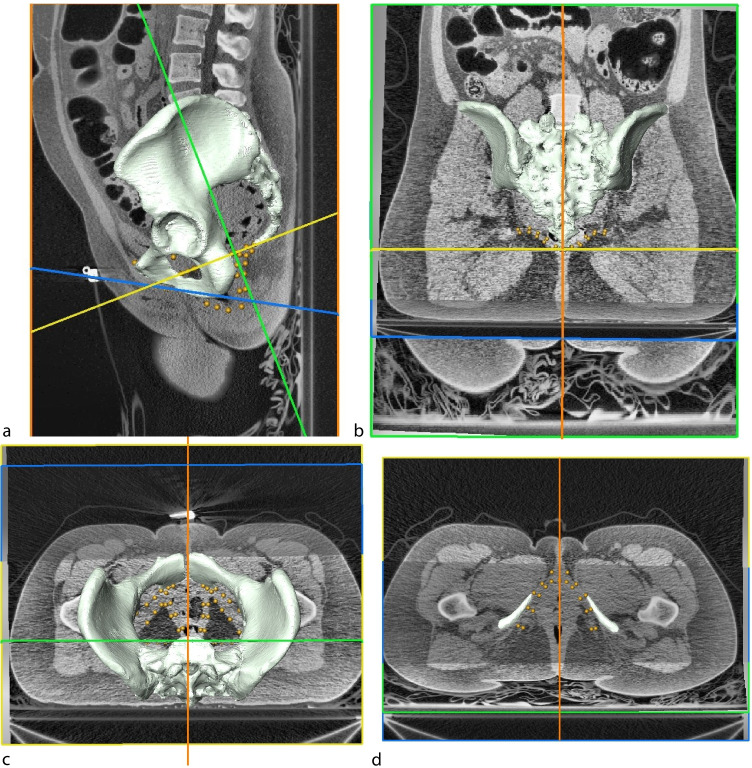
Slices used to place landmarks on outlines of pelvic floor muscles. The figure displays a 3D surface reconstruction of the pelvis from a CT scan and four slices through the CT stack used for collecting landmarks. *Orange* sagittal, *yellow* pubococcygeal, *green* iliococcygeal and *blue* ischiocavernosus slices. a Sagittal view; b posterior view; c superior view; d inferior view
